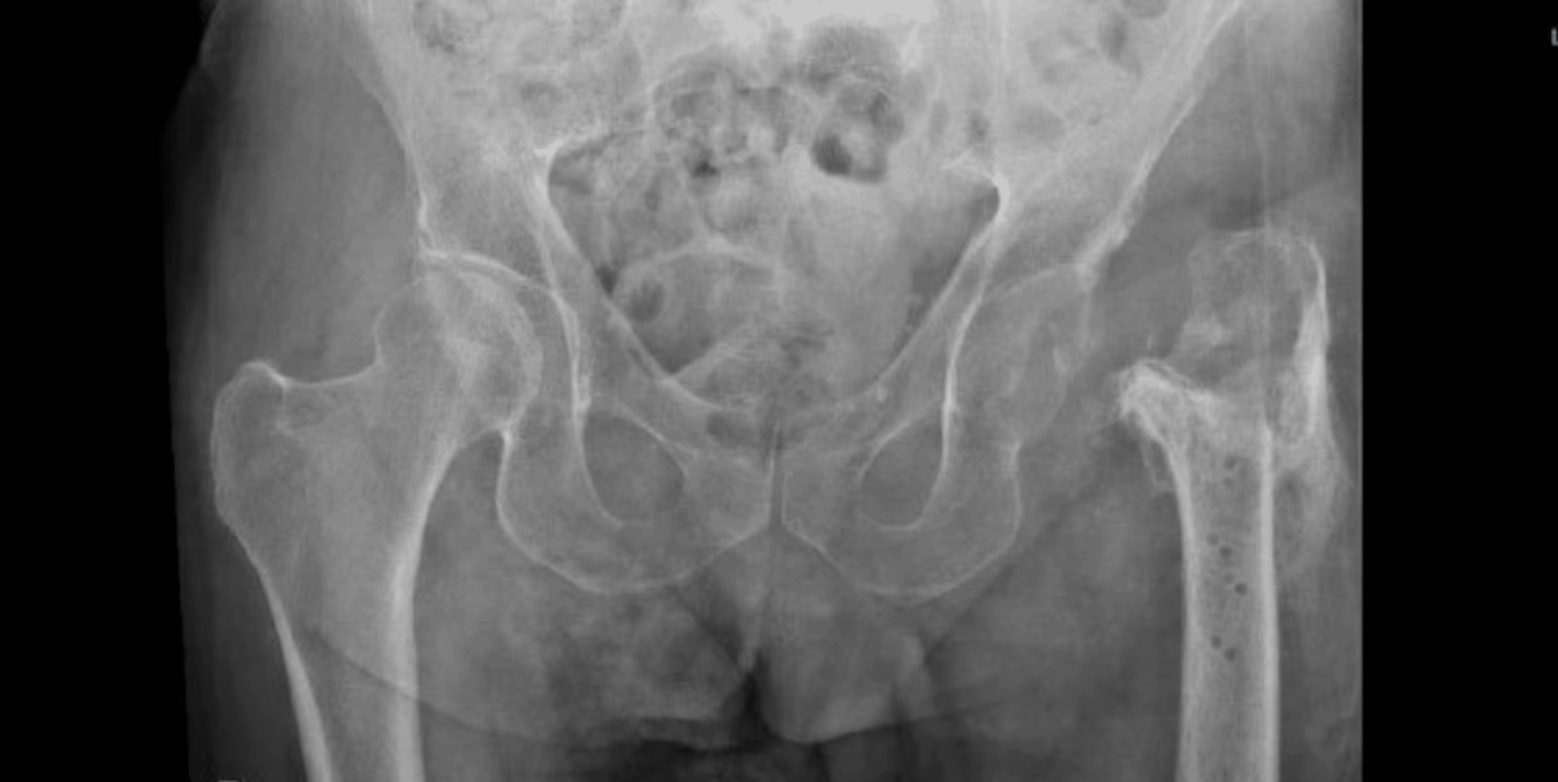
Pelvic X-ray anteroposterior view post-left hip excision arthroplasty
